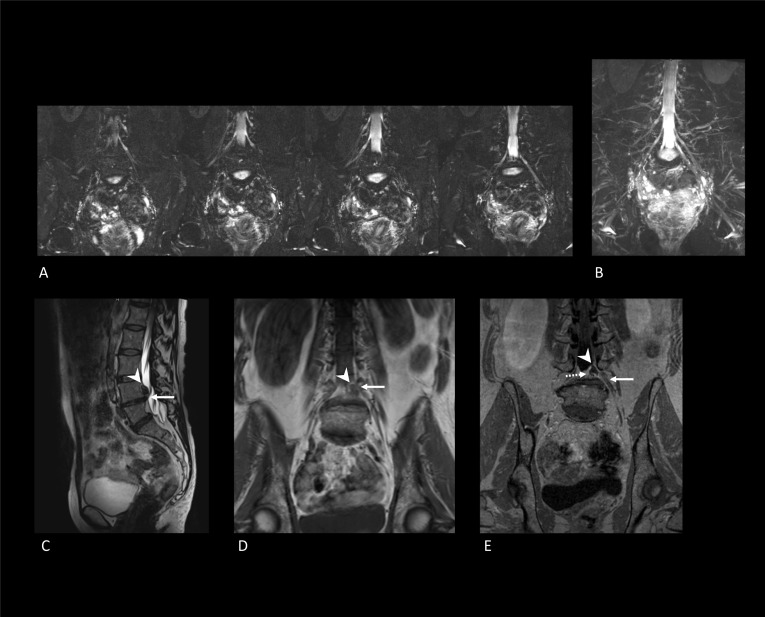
MR neurography examination of two FBBS patients. A Subsequent coronal 3D short tau inversion recovery sequence (3D STIR) images of one patient with no abnormalities. B Maximum-intensity projection of the 3D STIR images of the same patient (as depicted in A) showing no abnormalities of the lumbar plexus. C Sagittal T2-weighted MRI of a different patient showing a disk herniation occupying the left subarticular zone at level L4–L5 (white arrowhead). The white arrow depicts the coursing spinal nerve L4 on the left. D Coronal T1-weighted MRI of the same patient (as depicted in C, E) showing the disk herniation (white arrowhead) and left L4 spinal nerve (white arrow). E Coronal T1-weighted post-contrast MRI showing enhancing tissue (white dotted arrow) surrounding the disk herniation (white arrowhead). In addition, subtle perineural enhancement of the left L4 spinal nerve (white arrow) can be appreciated
